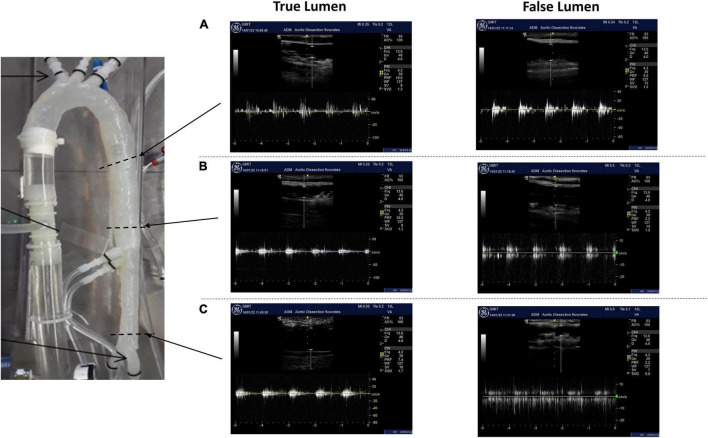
The average velocity profiles measured by Pulse Wave Doppler (PWD) along three sections of the aortic dissection model between (A) the first and second tears, (B) the second tear and superior mesenteric, and (C) the third tear and iliac vessels.
